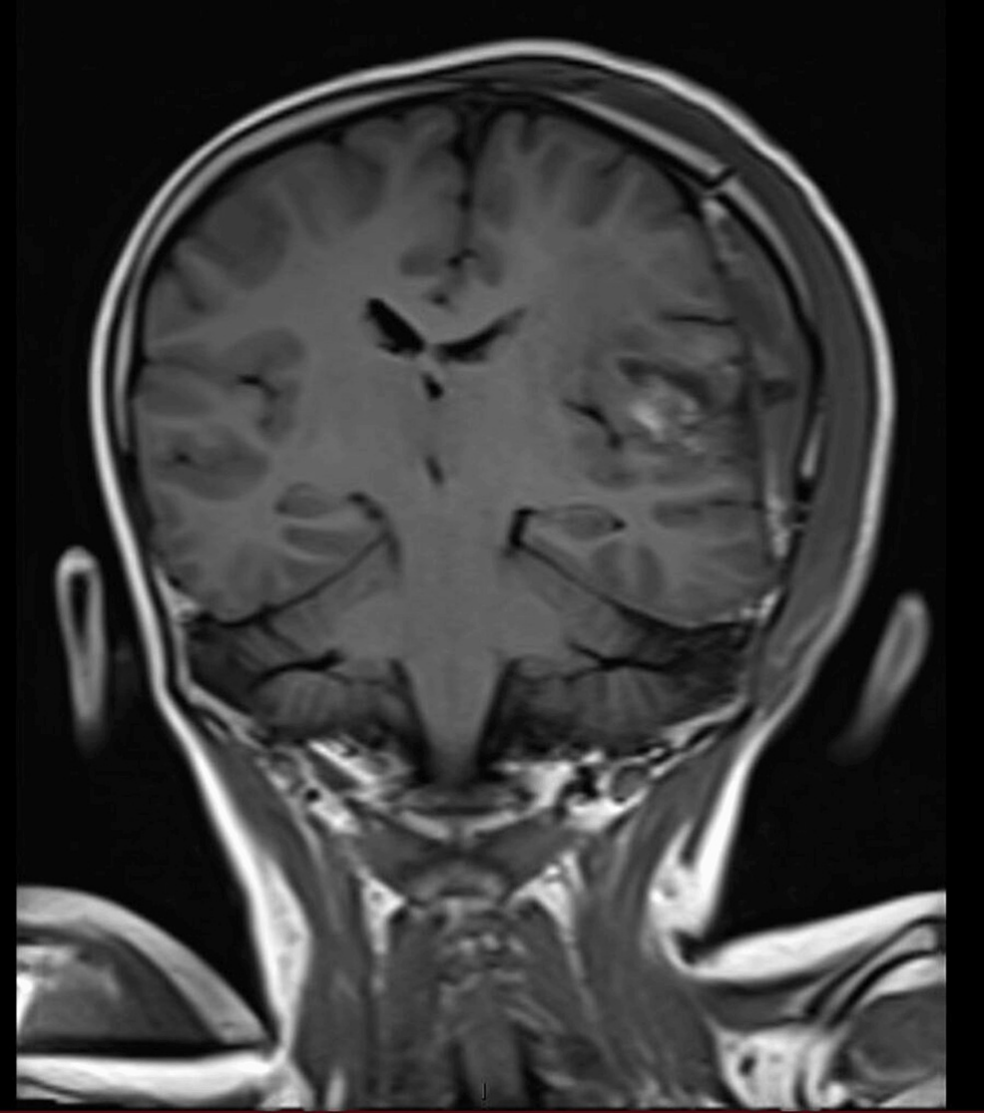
Postoperative magnetic resonance imaging scan in the T1 coronal view This scan does not show any residual tumor.
